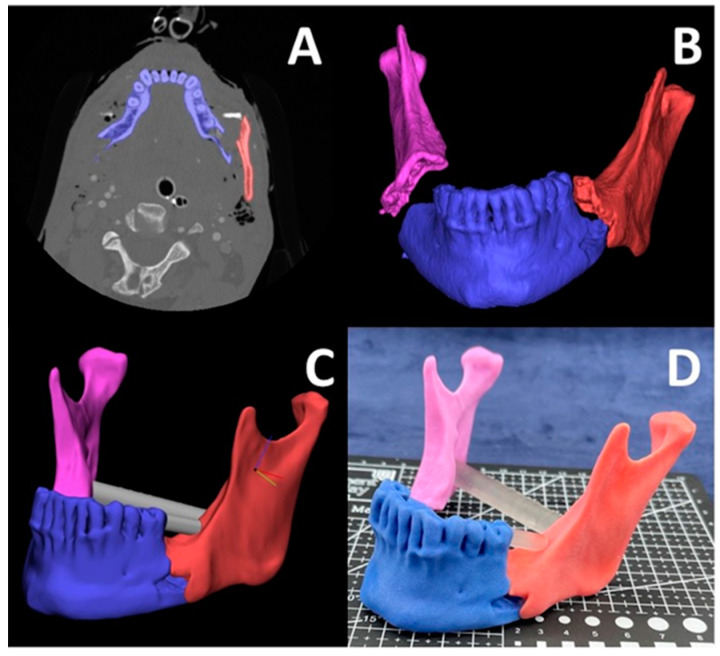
Bilateral open comminuted mandibular angle fractures requiring reduction with plating. (A) The axial CT scan with segmented masks of the symphysis (blue) and left angle (red). (B) 3D visualization of the segmented masks showing the bilateral mandibular angle fractures including the right mandibular angle (magenta). (C) The reduced 3D model in CAD software with pins for structural reinforcement, and (D) the final full color 3D printed using MJT technology.
